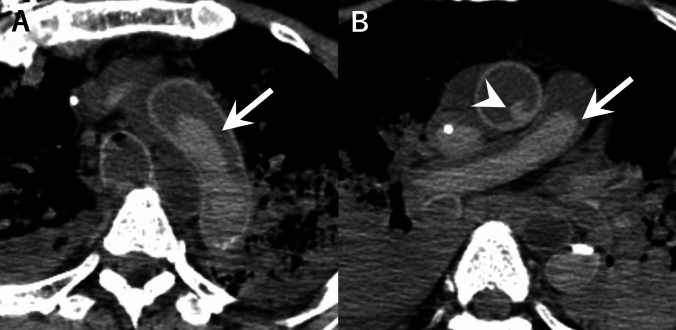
Example of a blood clot. A 64-year-old man with pancreatic carcinoma. A Axial postmortem CT showing cast-like blood clots (arrow) in the aortic arch. B Axial postmortem CT showing cast-like blood clot (arrow) in the pulmonary artery and ascending aorta (arrowhead). CT was performed 224 min after death
